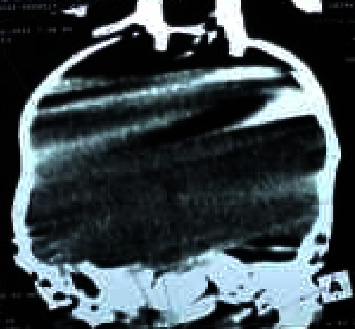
Parenchymal window head CT scan in coronal section showing 2 hyperdensities from either side the midline with metal artifact.
